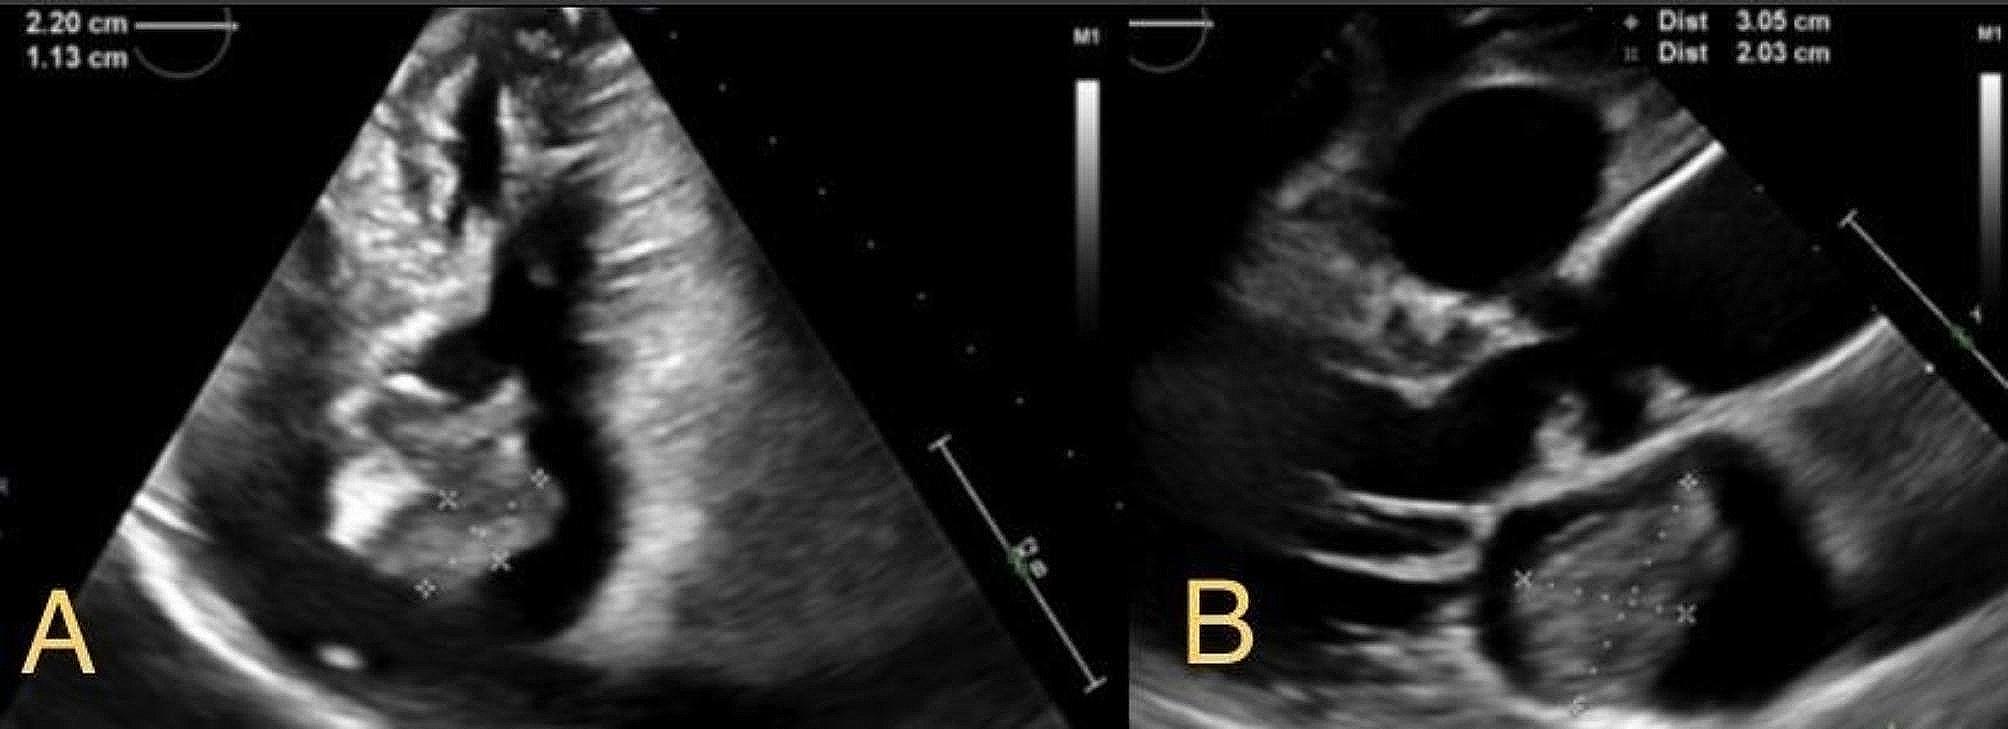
Transthoracic echocardiogram (TTE) images revealing a left atrial cardiac mass. A (apical 2-chamber): 2.2 cm X 1.1 cm on initial finding, B (parasternal long axis): 3 cm X 2 cm in 14-months from initial discovery
